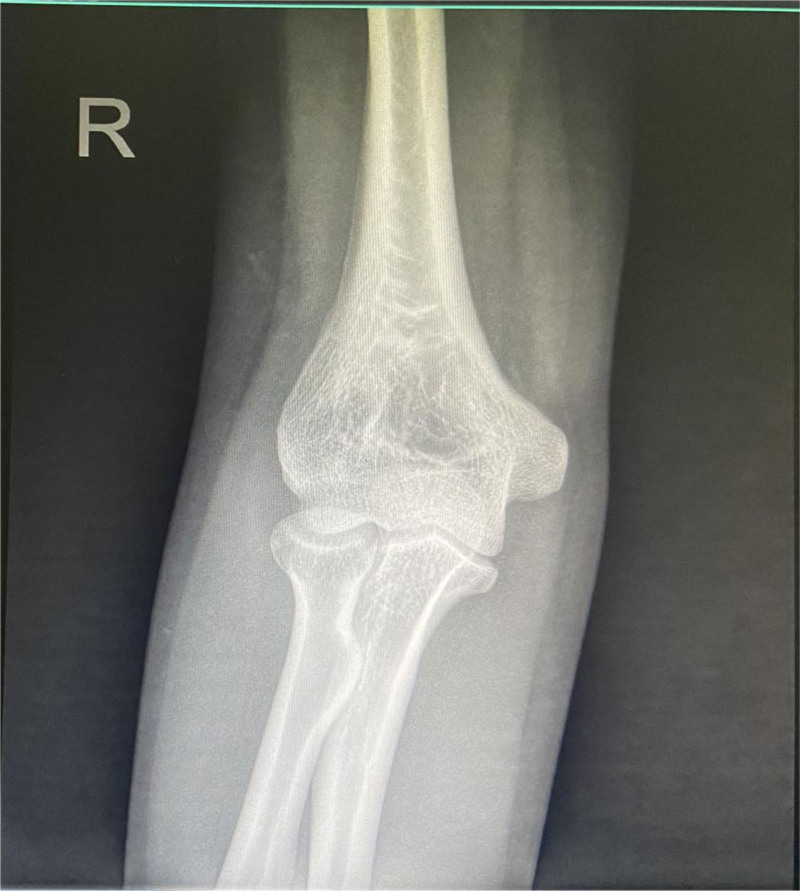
The postoperative plain radiograph showed a complete abolition of the heterotopic bony growth from the lateral epicondylar region.
